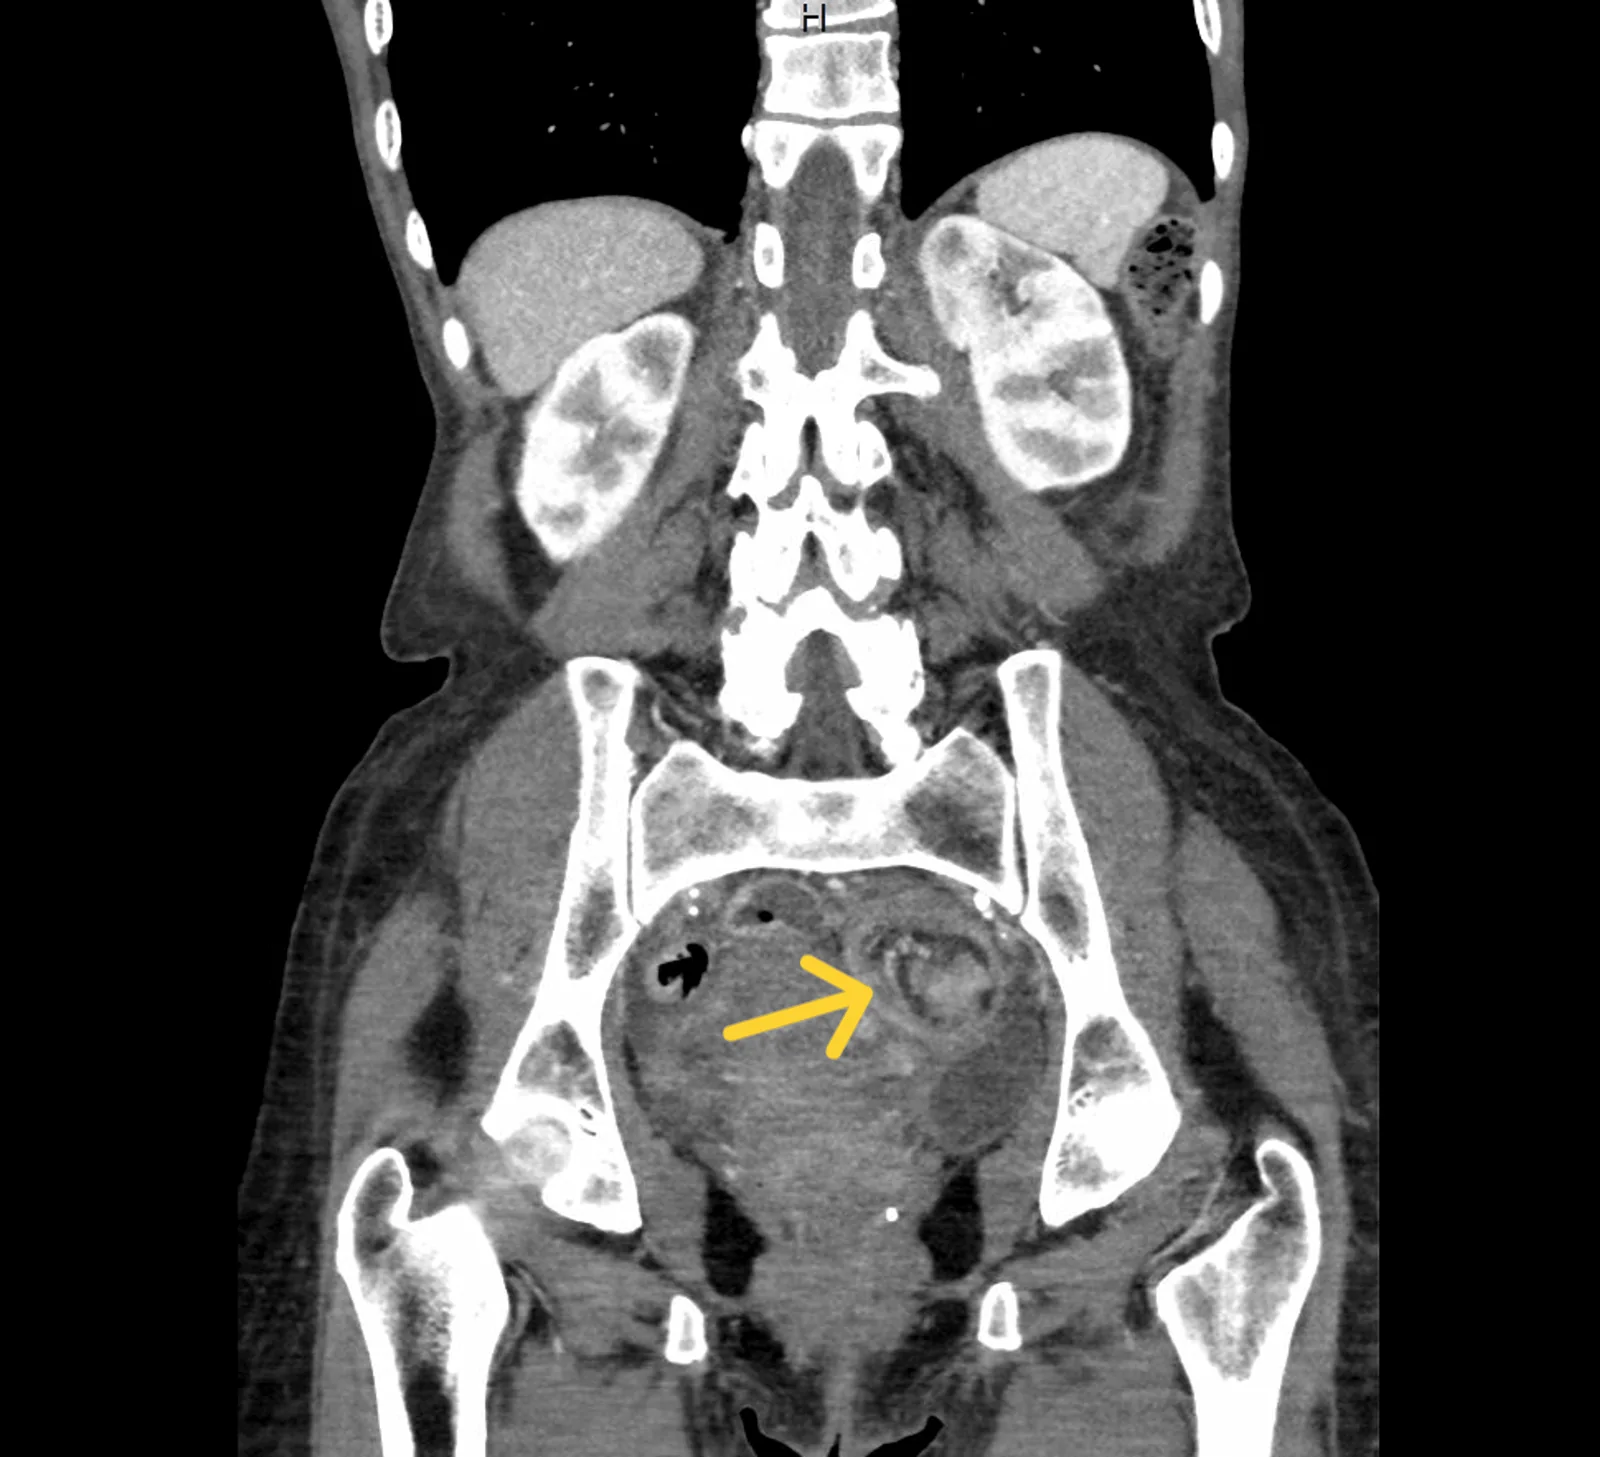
Target sign of rectosigmoid intussusception on CT
